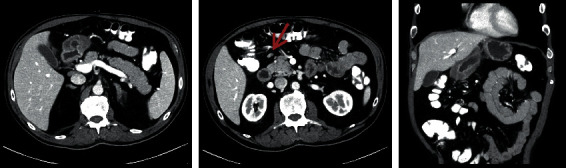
Computed tomography showing antral-wall thickening and mesenteric lymphadenopathy (arrow).
